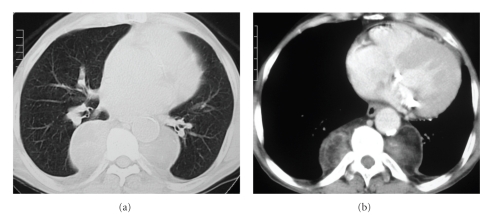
Computed tomography with pulmonary (a) and mediastinal (b) window settings demonstrates smooth heterogeneous (soft tissue and fat densities) bilateral masses in the paravertebral regions.
